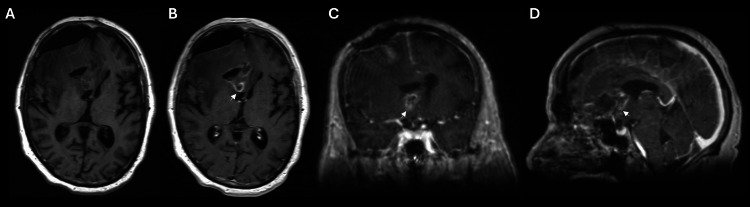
Post-operative MRI brain Post-operative MRI of the brain with axial T1 unenhanced image (A) demonstrates expected post-operative changes in the right frontal region, including expected pneumocephalus. The T1 post-contrast axial (B), coronal (C), and sagittal (D) images demonstrate a small amount of peripherally enhancing residual cystic lesion deep to the surgical site, extending into the remodeled and enlarged cribriform plate (arrowheads). Remodeling of the cribriform plate without destructive changes would suggest a relatively nonaggressive lesion, consistent with the pathologic diagnosis of epidermoid cyst. Mild leftward deviation of the right hemisphere is also present. MRI: Magnetic resonance imaging
